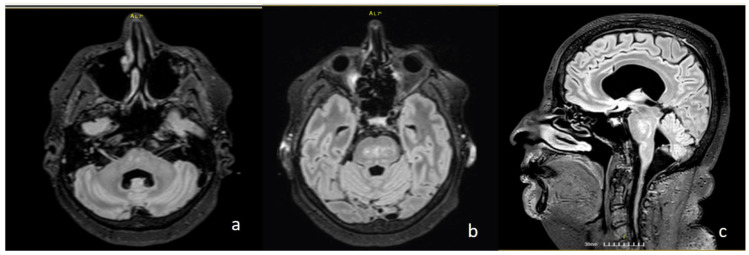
FLAIR images of the patient showing multiple non-enhancing lesions, mainly in the pons, (a) at the level of the vestibulocochlear nerve and nuclei, (b) at the level of the pons, and (c) sagittal view.
